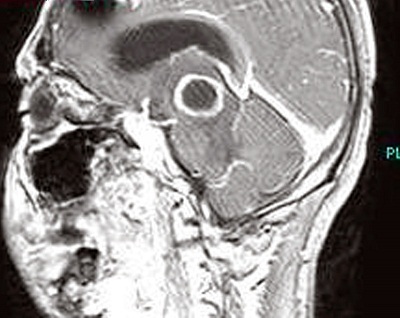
T1-weighted sagittal MR image with contrast showing a thalamic ring-enhancing lesion and artifact due to shrapnel in left frontal bone
